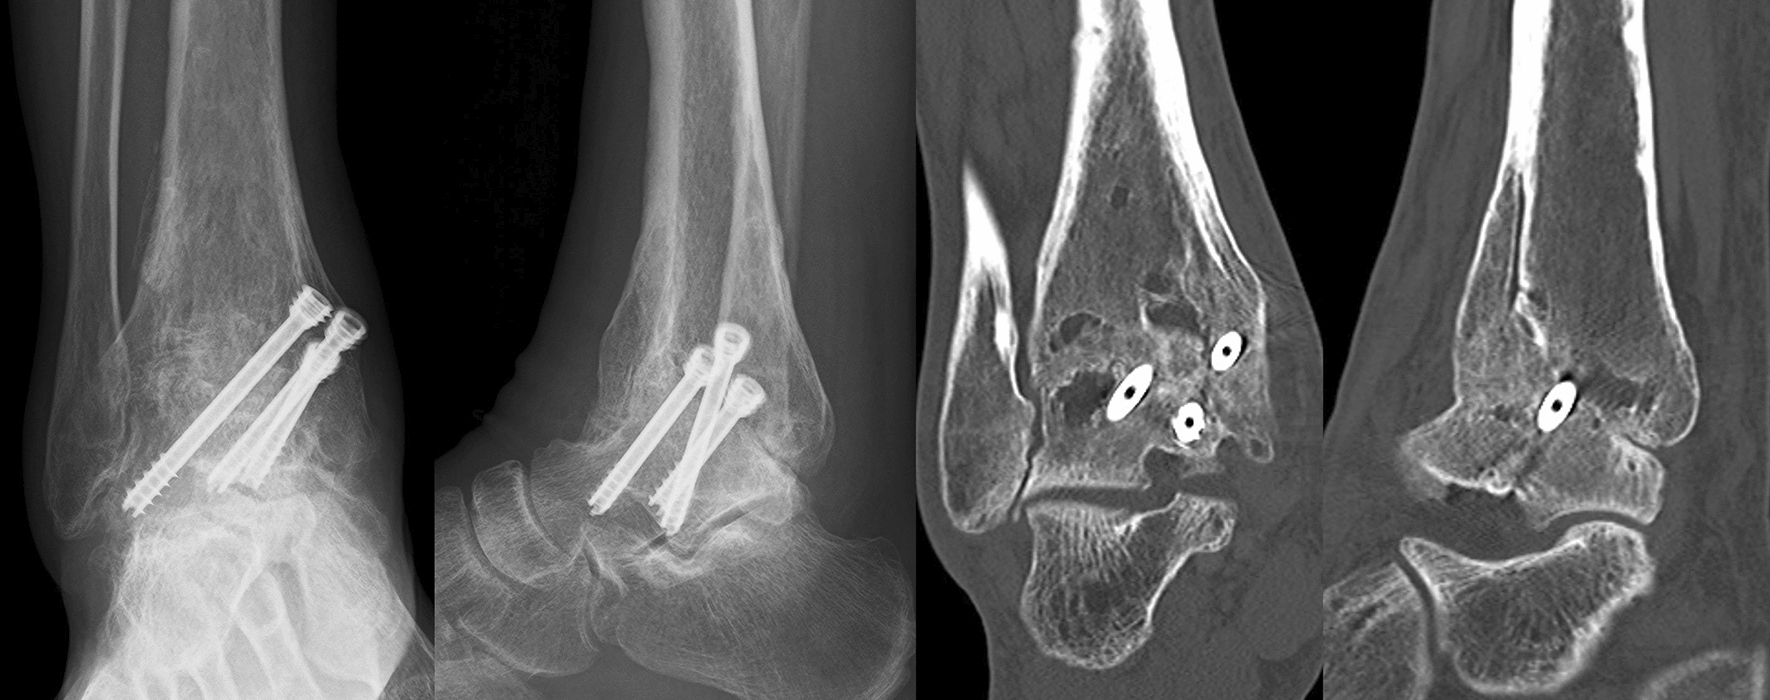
Radiological evaluation performed 2 years after the second stage, demonstrating complete consolidation after the ankle arthrodesis. Plain radiographs of the right ankle
